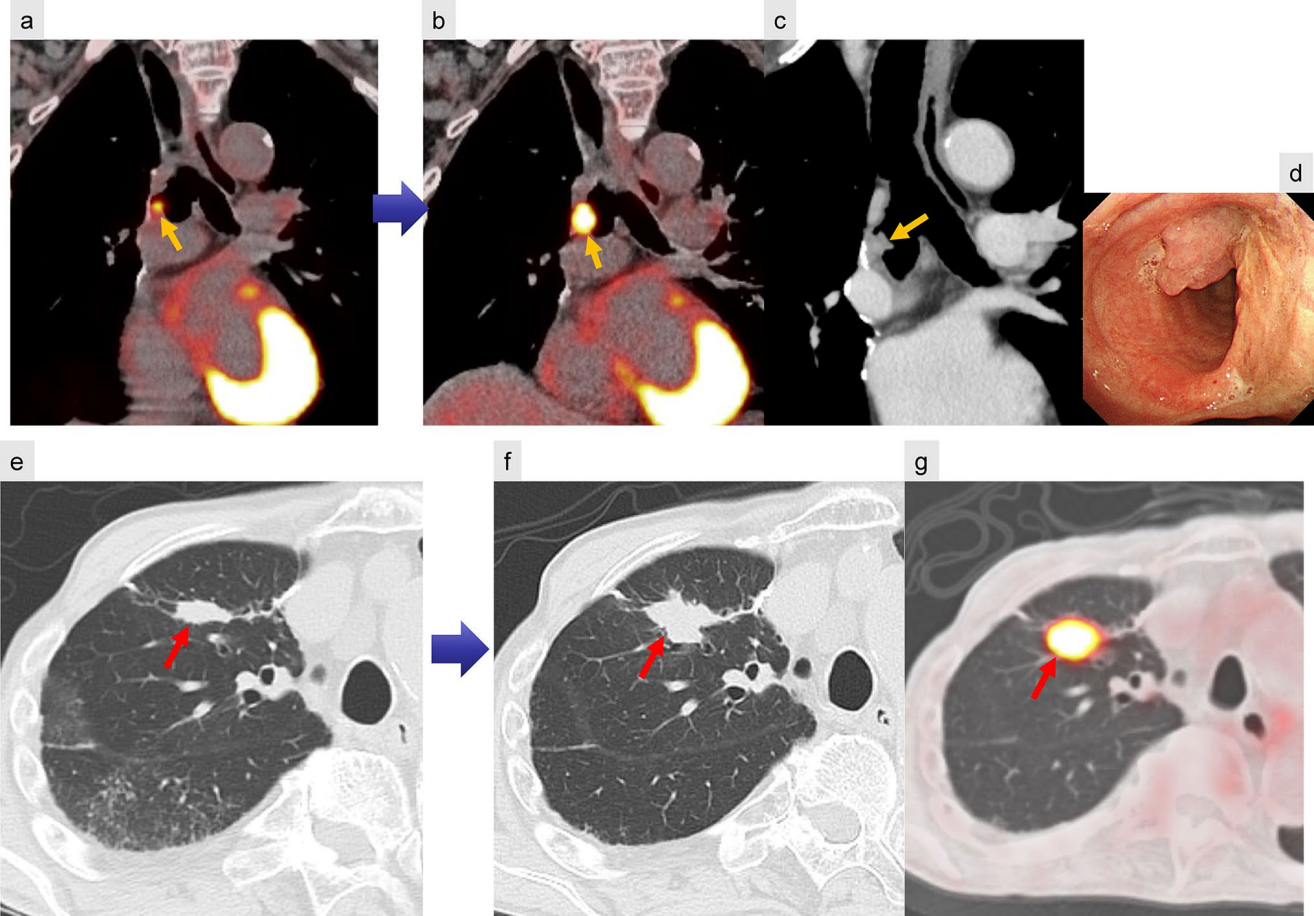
Local tumor recurrence. Bronchial stump recurrence after right upper and middle lobectomy (a–d). FDG-PET/CT performed 7 months postoperatively demonstrates increased FDG uptake in a small nodular lesion at the bronchial stump (a), which shows further progression at 13 months (b). CT reveals a soft-tissue mass protruding into the right bronchial lumen (c), and bronchoscopy confirms local recurrence (d). Staple line recurrence after partial resection of the right upper lobe lung cancer (e–g). Serial CT scans at 3 years (e) and 4 years (f) postoperatively demonstrate progressive soft-tissue enlargement along the staple line. FDG-PET/CT at 4 years shows abnormal FDG uptake consistent with tumor recurrence (g)
